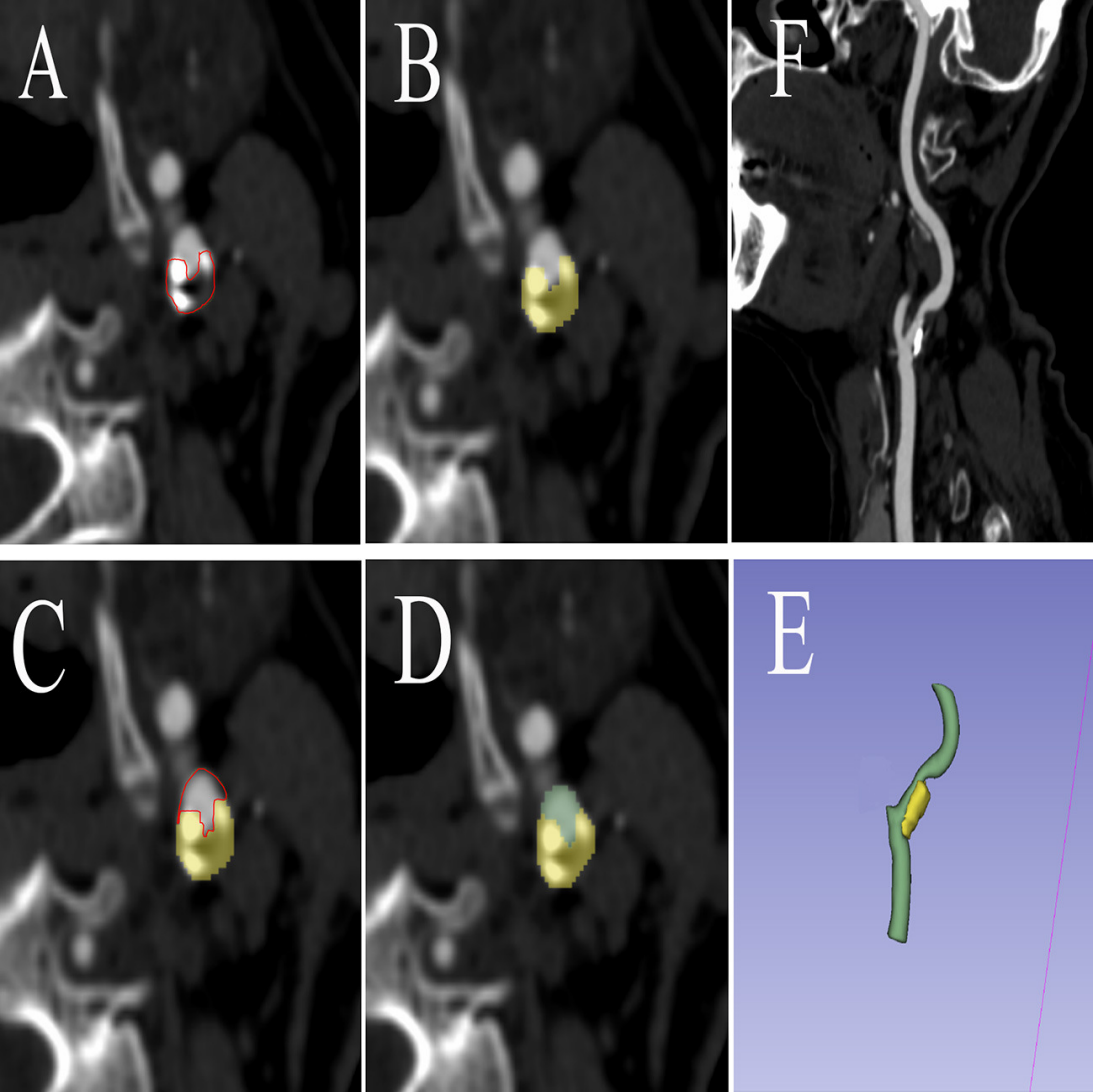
The procedure of radiomics segmentation. The VOI in each image was segmented with the green arterial lumen and yellow plaques, and a 3D model was synthesized. (A) The edges of atherosclerotic plaques were delineated. (B) Atherosclerotic plaques were segmented. (C) The edges of arteries were delineated. (D) Arteries were segmented. (E) A 3D model was built based on the segmentation of each layer image. (F) Carotid artery CTA corresponding image of the patient. VOI, volume of interest; CTA, computed tomography angiography.
